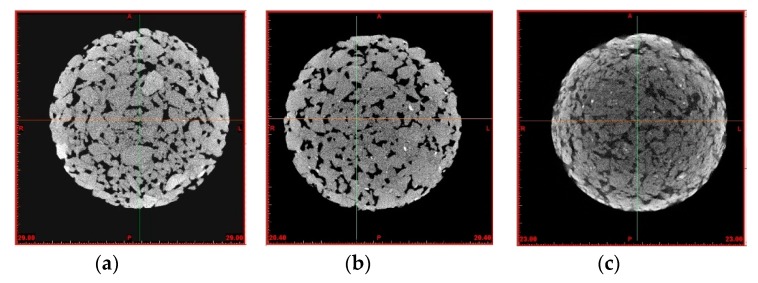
Computed tomography (a) PA-SCC; (b) PA-SLL; (c) PA-ELL.
